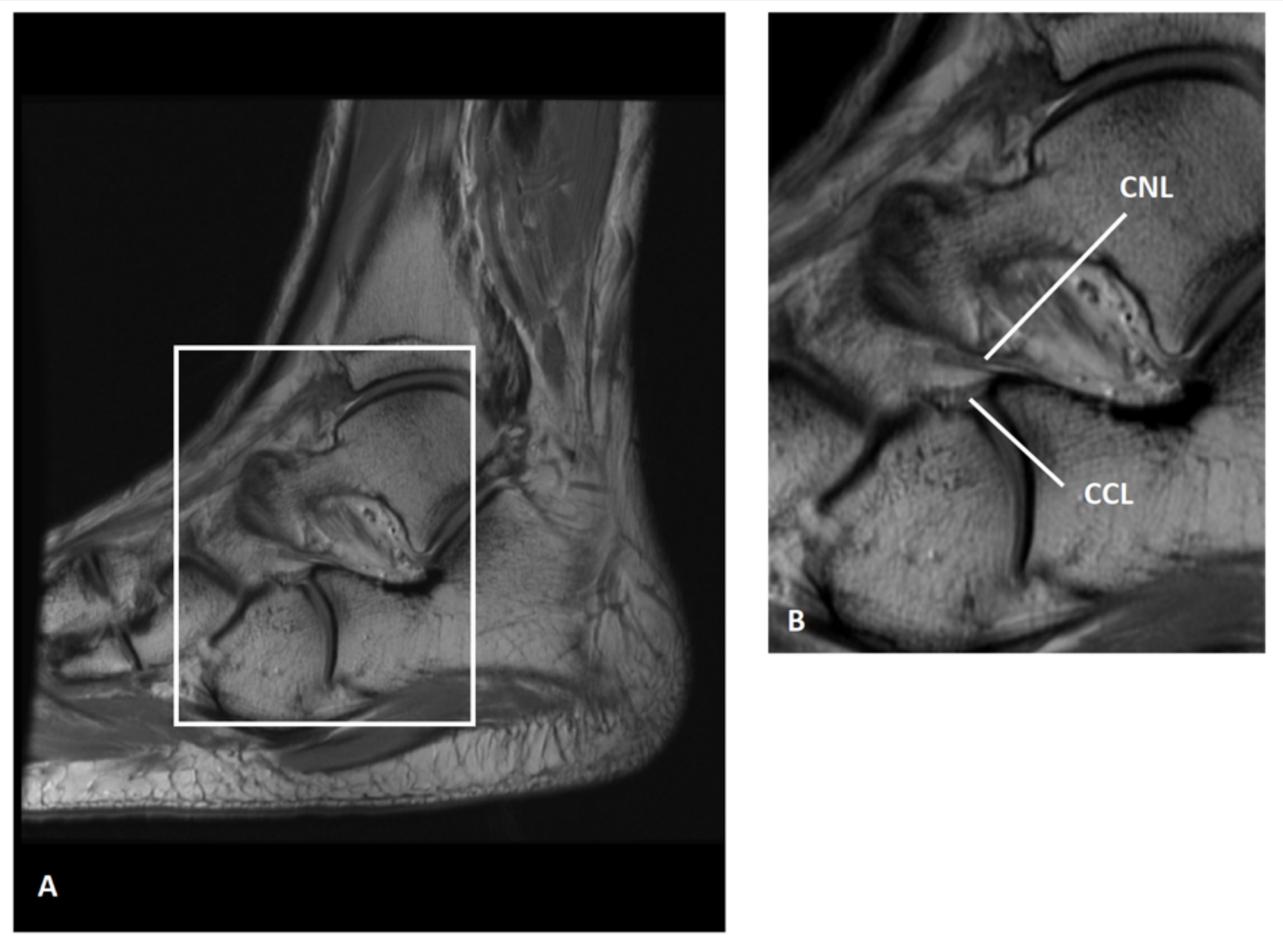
Bifurcate ligament of a 30-year-old man shown on a sagittal, proton-density magnetic resonance imaging Panel A, left foot with box delimiting the bifurcate ligament; Panel B, magnified view of the box shown in Panel A. (Images courtesy of Douglas N. Mintz, MD, FACR, Hospital for Special Surgery, NY) CNL: calcaneonavicular ligament; CCL: calcaneocuboid ligament.
